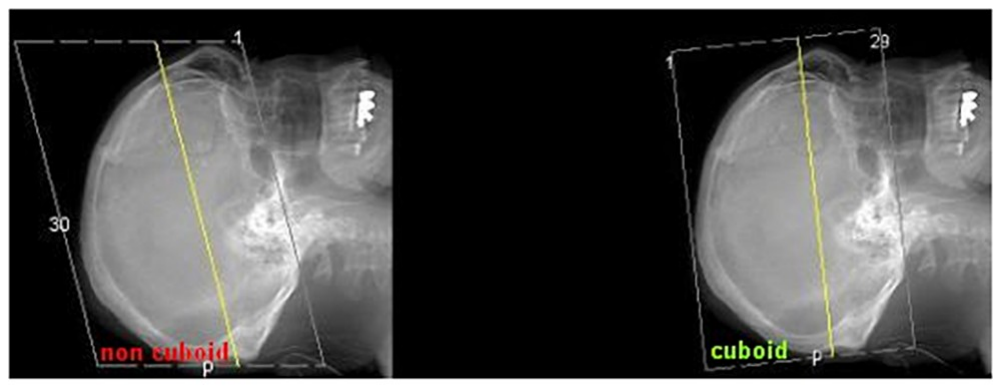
In order to be able to apply Pythagoras’ theorem, the line “travelled” by the cursor through the slices must be vertical to the slice orientation. This is only the case if the slices are “piled aligned” in a cuboid dataset (*right*). If the gantry angle is modified during data acquisition, the resulting dataset is a non-cuboid parallelepiped, and the calculation cannot be applied. In that case, a cuboid dataset can be reconstructed at the CT workstation. Whether the regarded slices are applicable for the caliper navigation technique or not can be seen at a glance in the corresponding scout.
